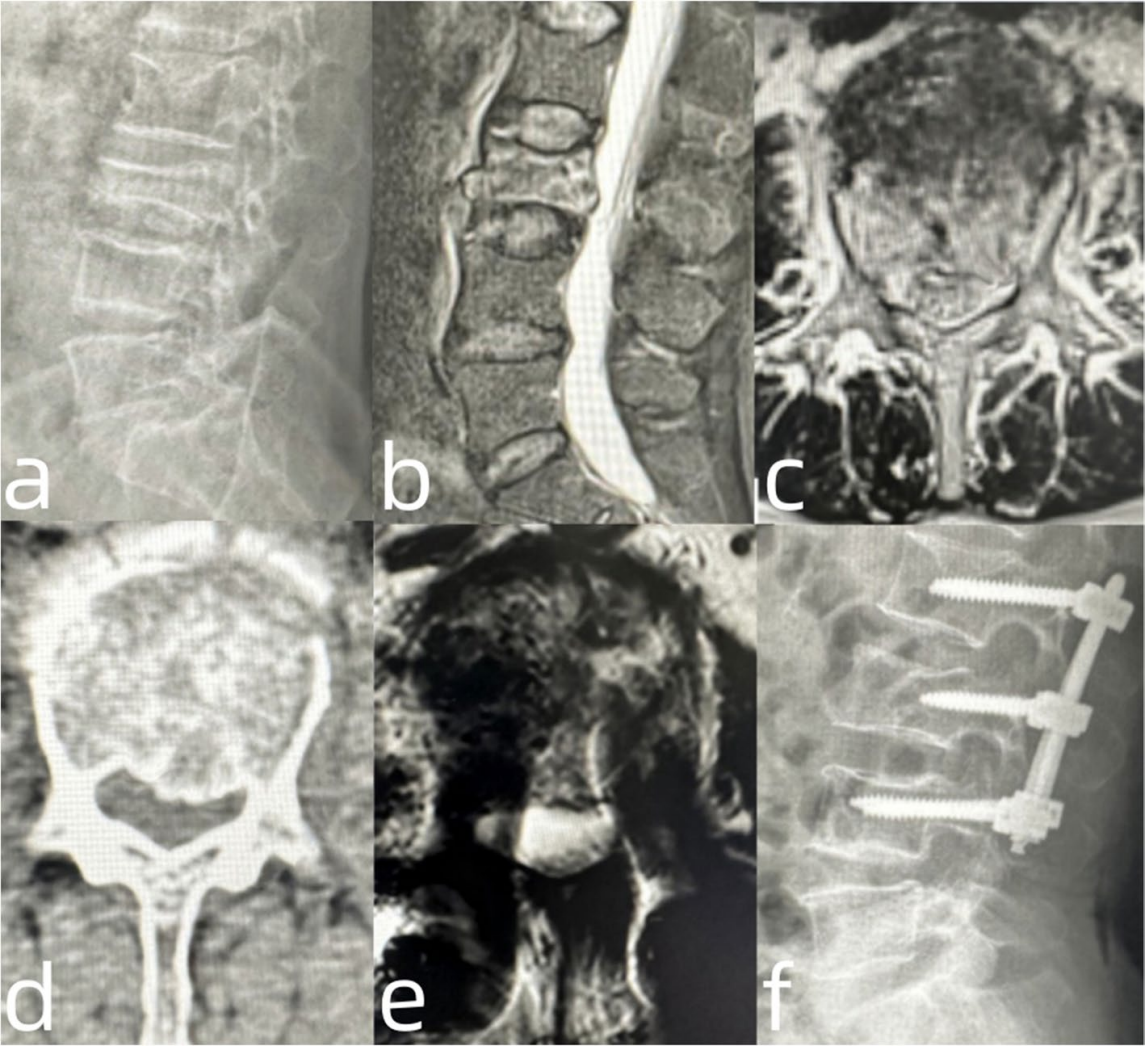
a The lateral view of the preoperative radiography of lumbar spine showing that the L3 vertebral height was significantly decreased and the lumbar lordosis was lost; b, c, d: Preoperative MRI and CT of lumbar spine manifesting a burst fracture of L3 vertebral body and encroachment of the central canal and left lateral recess on L3 vertebra; e: Postoperative cross-sectional MRI showing a well decompression of the central lateral and lateral recess; f: Postoperative lateral radiography showing a satisfactory reduction of L3 vertebral body height and an increase in lumbar lordosis
